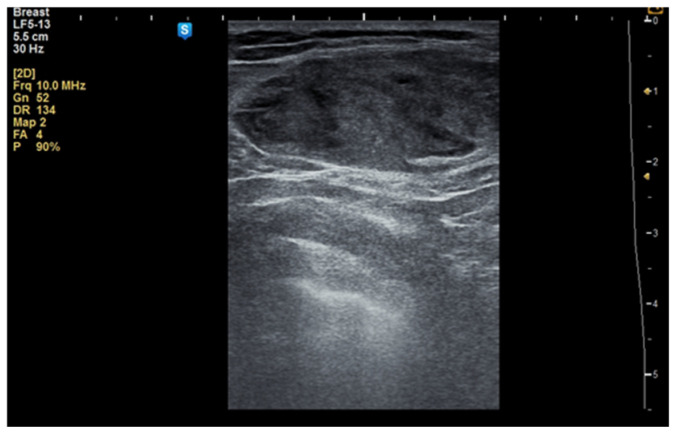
Ultrasound image of a 34-year-old woman with a right breast hamartoma of 65/35 mm, with heterogeneous hypoechoic structure, located in the upper inner quadrant.
